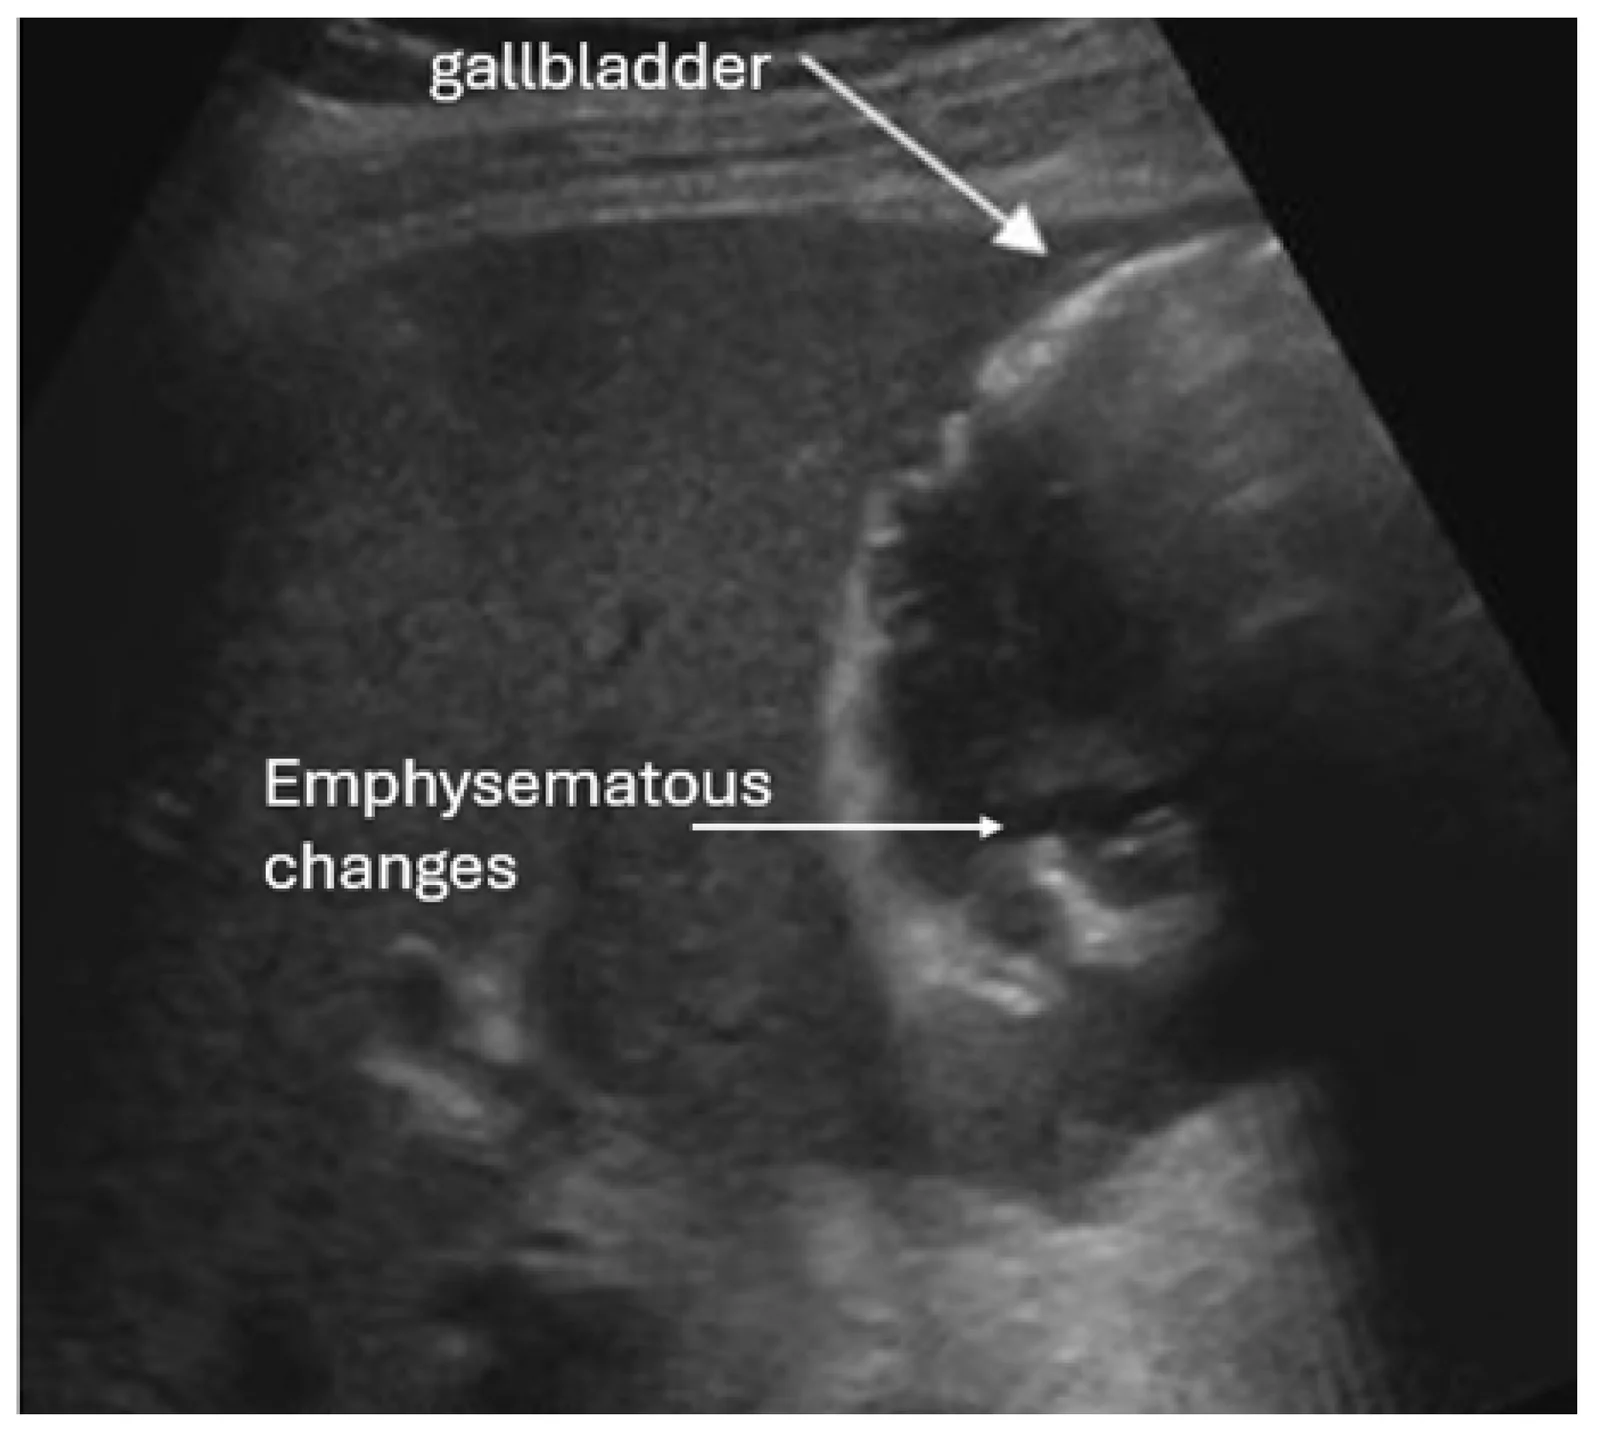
Another serious gallbladder complication is emphysematous cholecystitis, caused by bacterial superinfection of the gallbladder wall with gas-producing organisms. Diabetes mellitus with hyperglycemia is a significant risk factor, and the gallbladder can become ischemic with poor vascular flow [3,4]. Ultrasound characteristics include presence of air within the gallbladder wall, which appears as hyperechoic foci with associated reverberation or “dirty shadowing” artifact [3,4,13,14,15]. Ultrasound is highly specific for detecting air within the gallbladder wall, though sensitivity is decreased due to air interfering with clear visualization of the gallbladder [13,14,15]. Figure 4 is an example of emphysematous cholecystitis with free air within the gallbladder wall and posterior “dirty shadowing”. A CT scan should be performed if ultrasound images are not clear. When emphysematous cholecystitis is diagnosed, physicians should emergently consult general surgery and initiate intravenous antibiotics.
